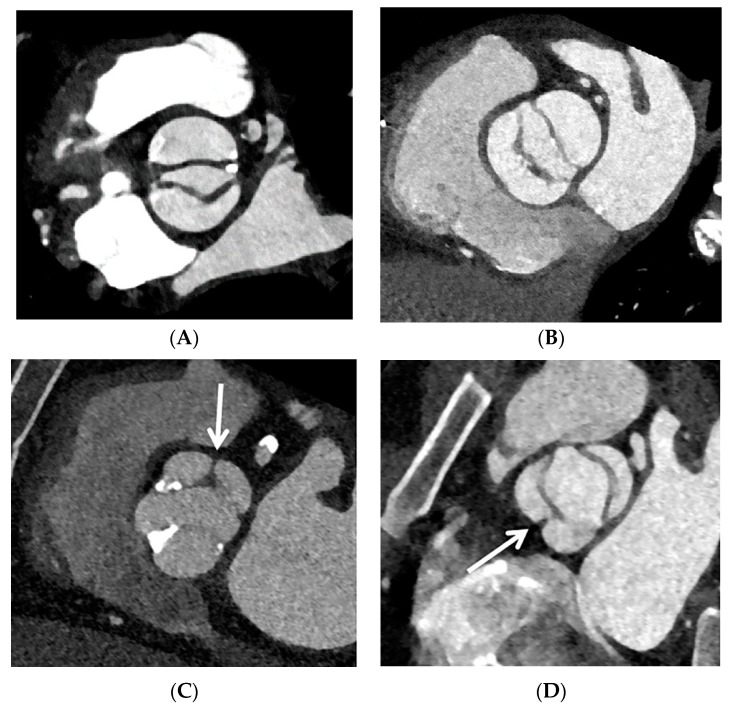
Bicuspid aortic valve classification: valve type according to the absence (A,B) or presence (arrow, C,D) of a raphe on cardiac CT in double-oblique short-axis plane in the systole.
